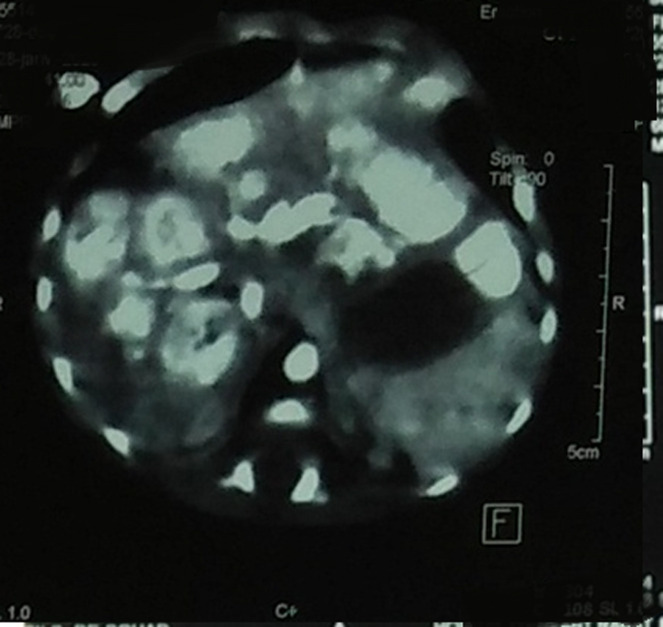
abdominal computed tomography cross-section: arteriovenous shunts
